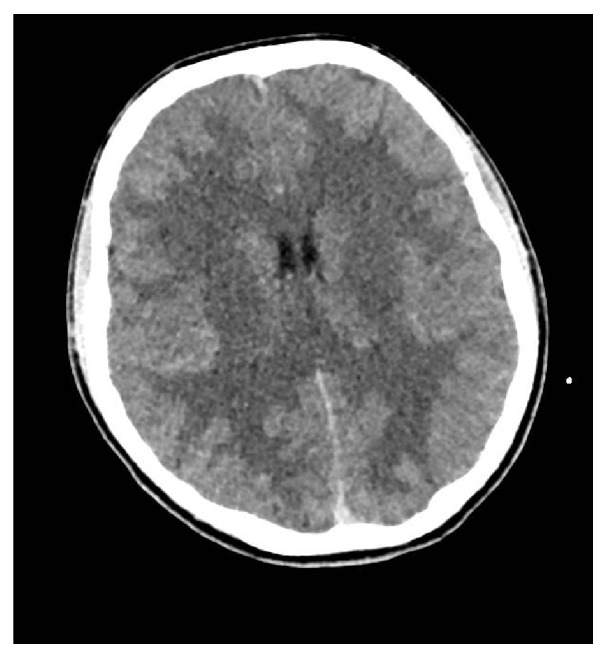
Cranial computed tomography (CT) scan performed at the end of the surgery: brain swelling.
